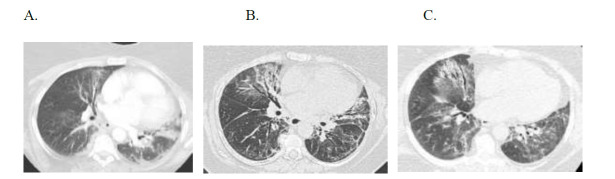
Case 3: Temporal evolution of fibrotic nonspecific interstitial pneumonitis in a 66-year-old female with an overlap of primary Sjogren’s syndrome and anti-synthetase syndrome. Axial HRCT images in (A) April 2017, (B) August 2017, and (C) September 2018 demonstrate basilar predominant ground glass opacities which evolved to include bronchiectasis and septal lines in the areas of ground glass four months, later indicating evolution from a primarily inflammatory process to fibrosis. 13 months later, there has been a further increase in the extent of disease with a larger percentage of abnormal lung and new ground glass opacity.
